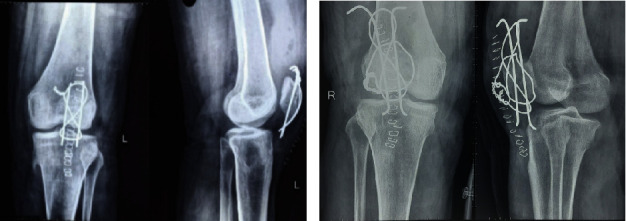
(a) Postop X-ray of the left patella. (b) Postop X-ray of the right patella.
